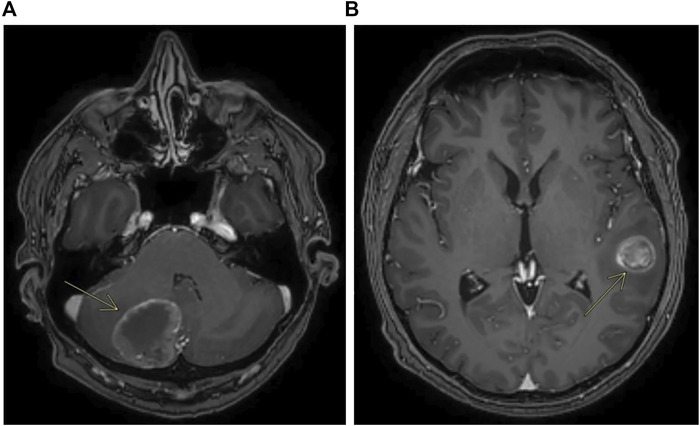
Contrast enhanced MRI showing metastases of the cerebellum (A) and metastases of the left temporal lobe (B).
